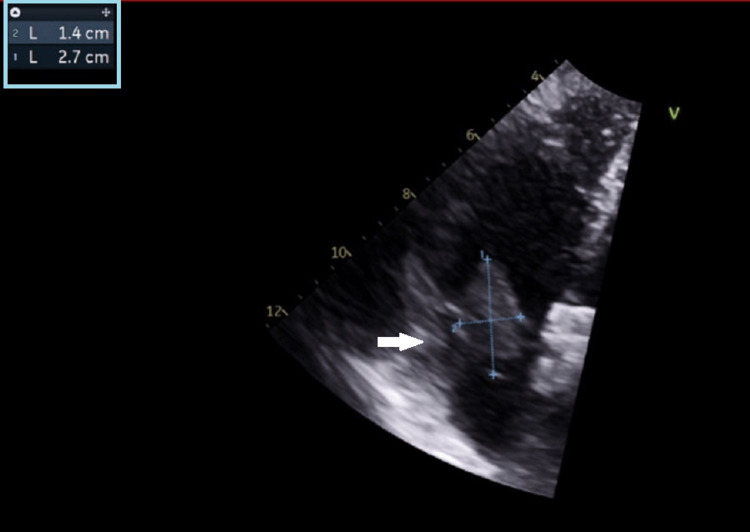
Transthoracic echocardiogram showed the size of the right atrium thrombus.
